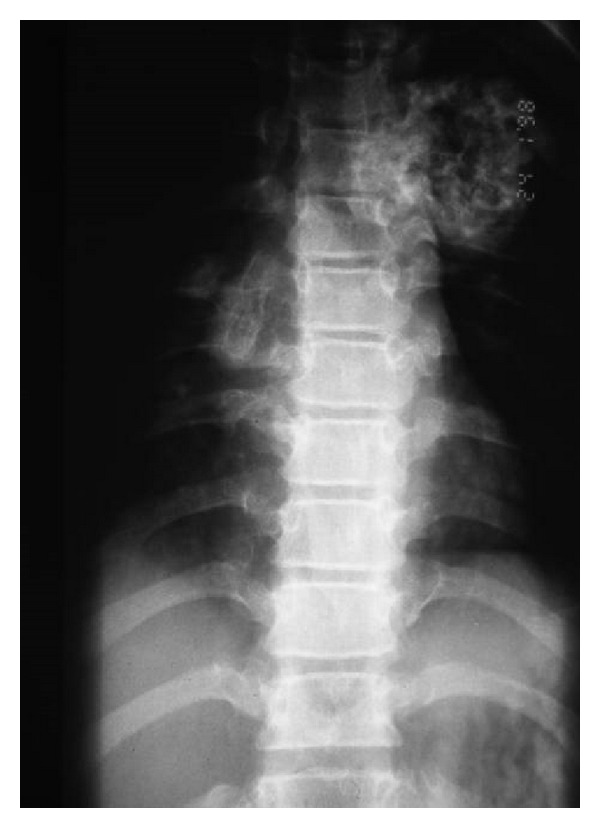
Conventional radiograph showed large cauliflower mass projecting along the anterolateral aspect of the spine.
